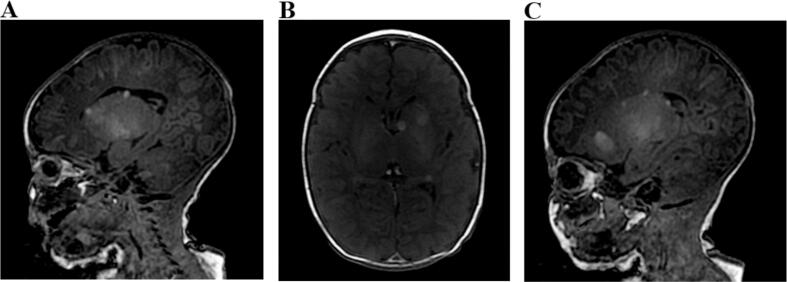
Brain MRI findings consistent with tuberous sclerosis complexFig. 1: T1-weighted brain magnetic resonance images demonstrate multiple hyperintense nodules along the ventricular lining, consistent with subependymal hamartomas (A), including an approximately 6-mm lesion near the left foramen of Monro (B). A focal T1-hyperintense lesion in the subcortical white matter is also shown, compatible with a cortical/subcortical tuber (C).
